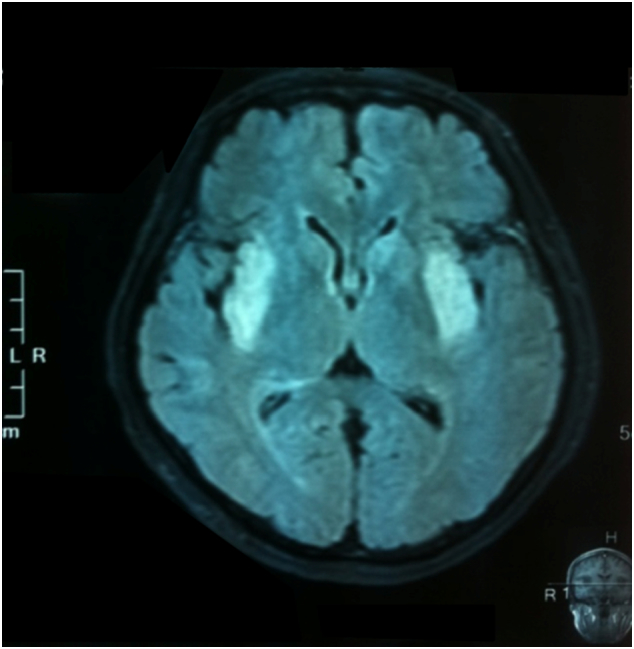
FLAIR MRI demonstrating bilateral contrast hyperintensity in the medial temporal lobes in a 44 year old male with anti-NMDA receptor encephalitis.
